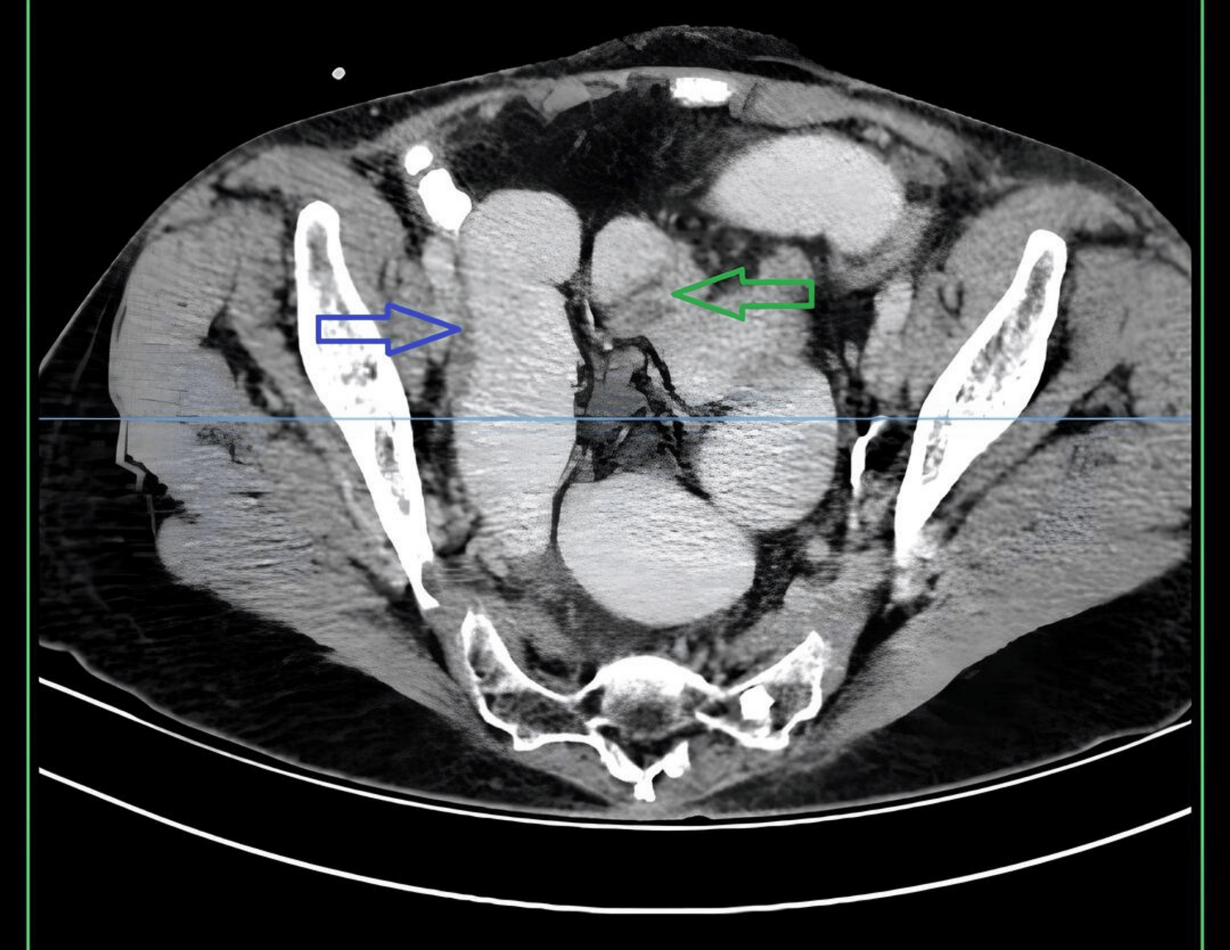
CT showing dilated loops of the small intestine in the pelvic cavity (blue arrow) and point of luminal narrowing of the right distal ureter (green arrow)
